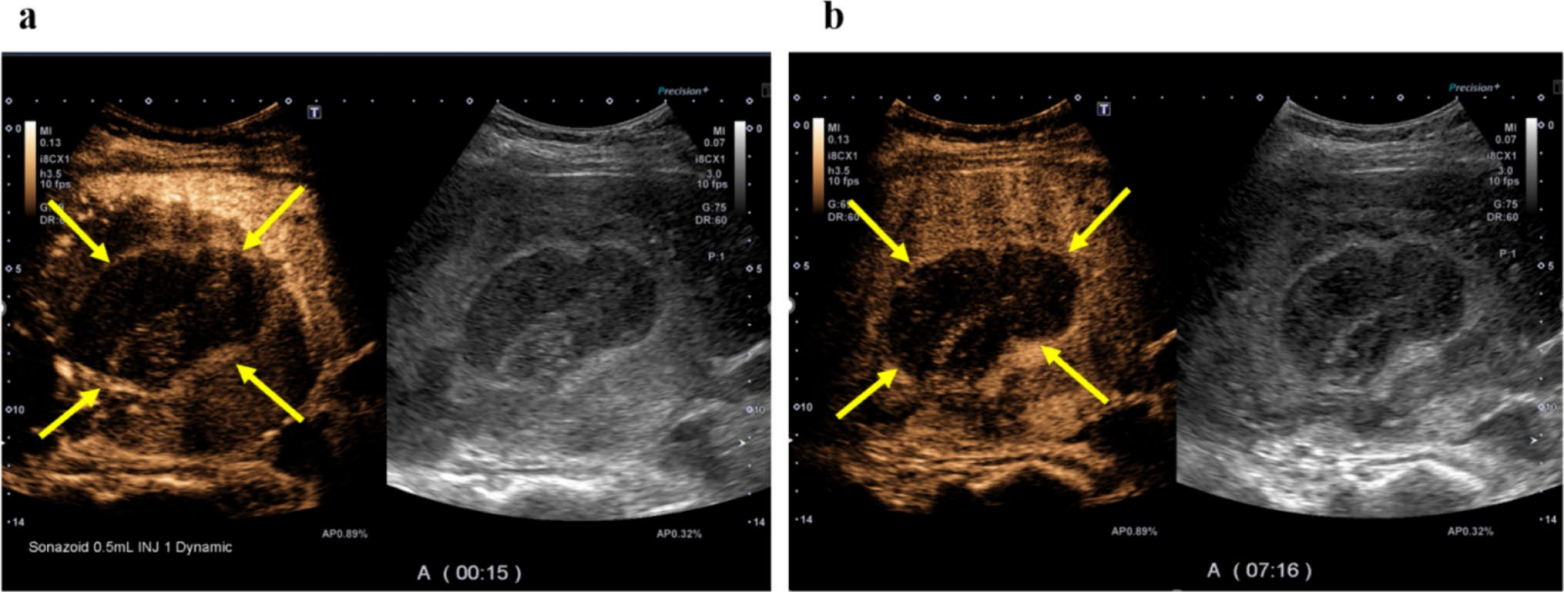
Abdominal contrast-enhanced ultrasound. a Vascular imaging and b Kupffer imaging. The arterial phase after injection of ultrasound contrast medium showed a fill-in pattern with contrast from the periphery of the hemangioma. Kupffer imaging showed a well-defined, non-contrasted area inside the hemangioma. The yellow arrows indicate it
